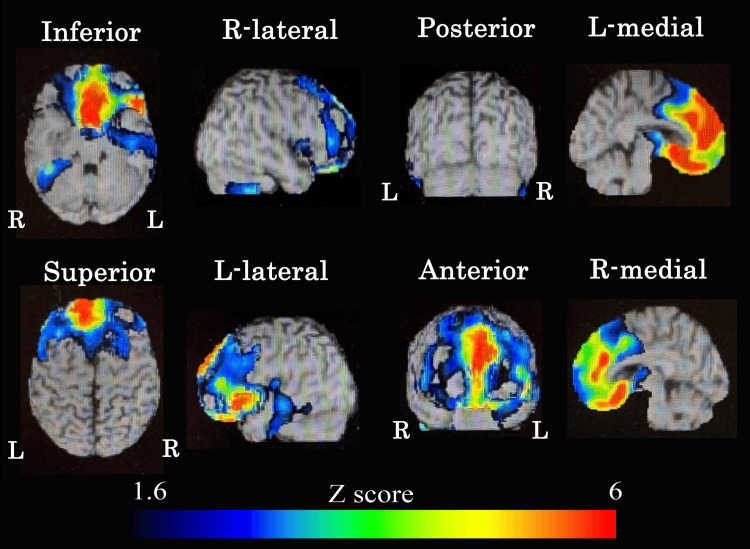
Single-photon emission computed tomography (SPECT) findings. ^99m^Tc-ECD SPECT imaging showing hypoperfusion in the left frontotemporal and limbic regions, with relative sparing of the posterior cingulate cortex and precuneus. ECD: ^99m^Tc-ethyl cysteinate dimer.
